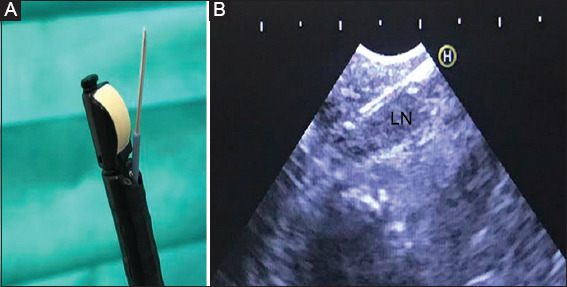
(A) Convex transducer of endobronchial ultrasound and transbronchial needle aspiration in the work channel. (B) Real-time of endobronchial ultrasound and the needle puncture of lymph node.
